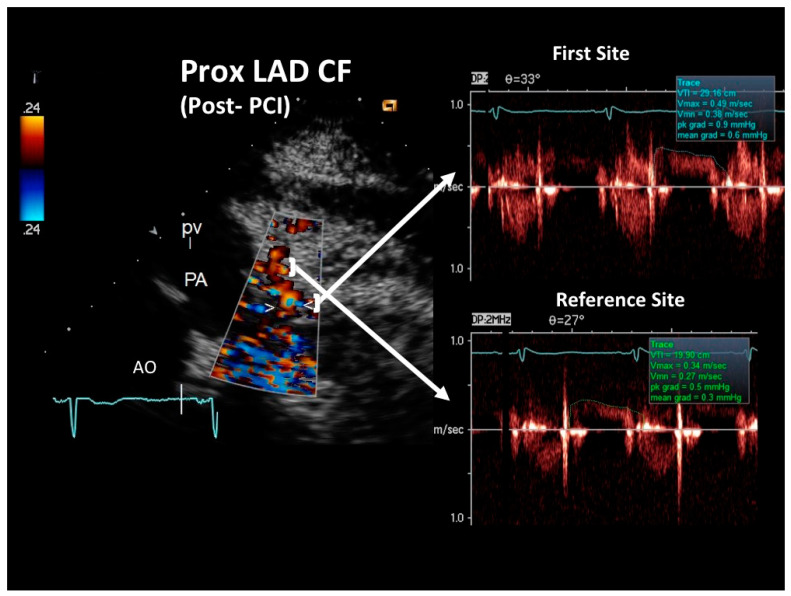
Blood flow Doppler recording by E-Doppler TTE in the proximal LAD after stenting in the follow-up (same format as in the acute phase). Maximal velocity had dropped to 49 cm/s, yielding a residual stenosis area of 25% according to the continuity equation. The diastolic waves of the Doppler tracings are outlined in green, and the maximal velocity and time velocity integral are reported. Abbreviations as in Figure 2.
